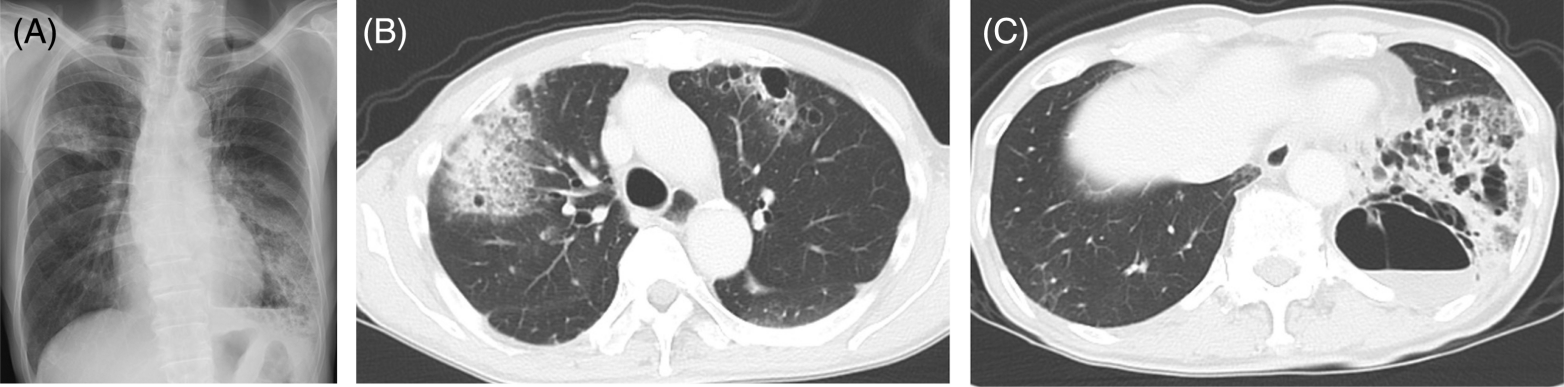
Chest x‐ray at initial examination (A). Chest computed tomography scan showing infiltrating shadow and bronchiectasis in the right upper lobe (B) and infiltrating shadow and cavitary lesions in the left lower lobe (C).
